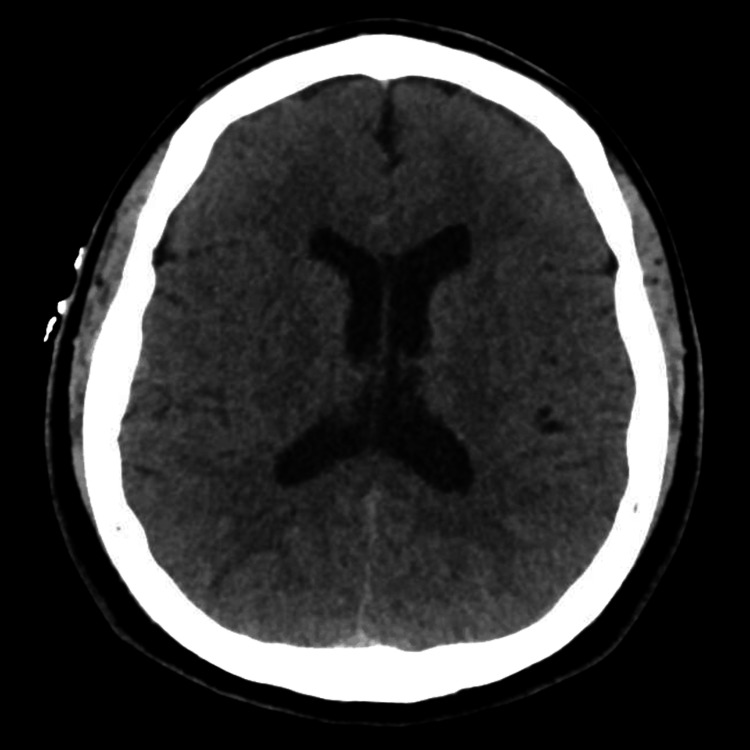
Computed tomography scan of the head showed no abnormalities.
